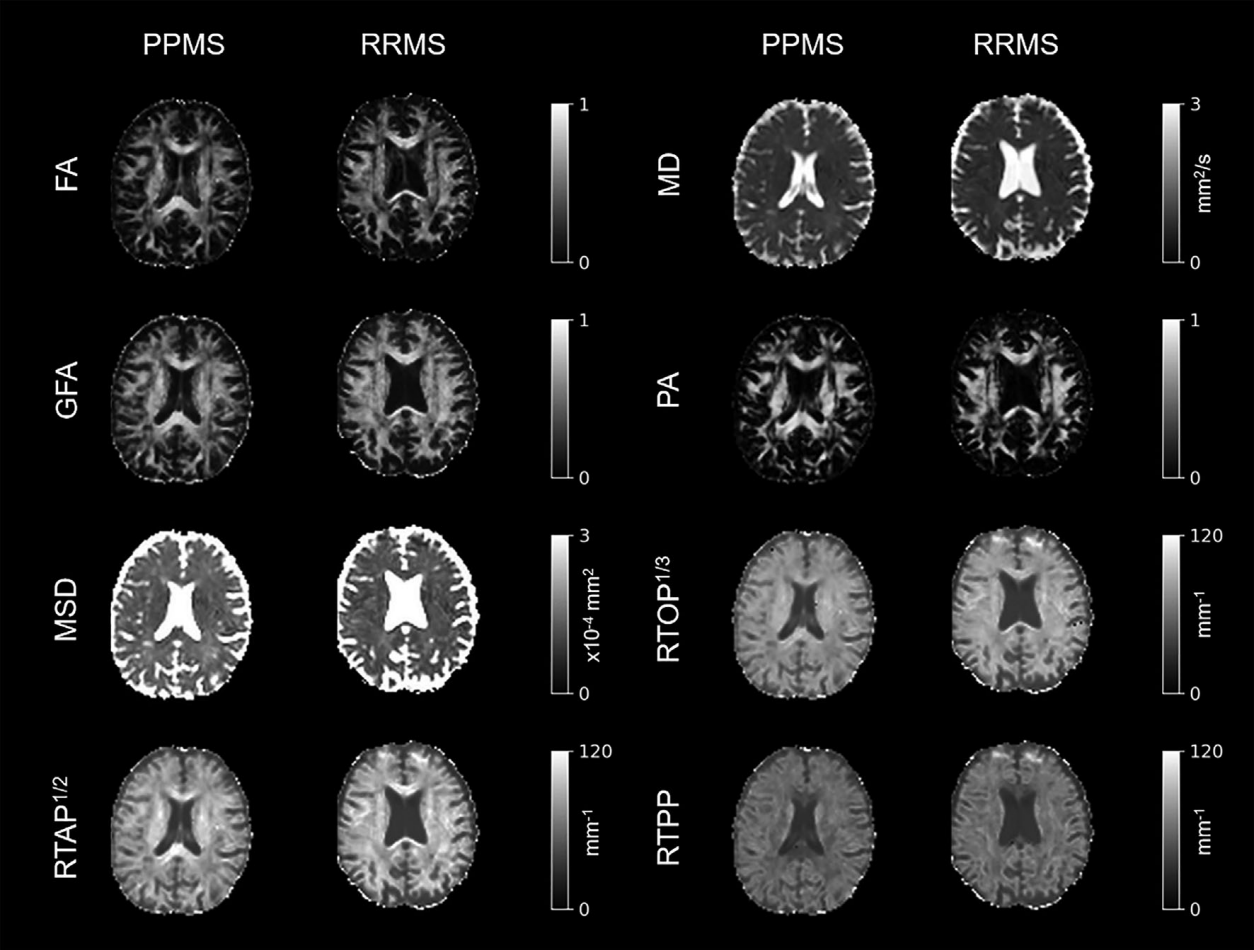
Microstructural indices from diffusion MRI (dMRI). For ease of comparison across RTOP, RTAP, and RTPP maps, the cubic‐ and square‐root of RTOP and RTAP were displayed. Axial slices of two representative patients (one primary progressive and one relapsing–remitting multiple sclerosis [PPMS and RRMS]) are reported for all microstructural indices. Images are displayed in radiological convention. FA = fractional anisotropy; MD = mean diffusivity; GFA = generalized fractional anisotropy; PA = propagator anisotropy; MSD = mean square displacement; RTOP = return to the origin probability; RTAP = return to the axis probability; RTPP = return to the plane probability.
